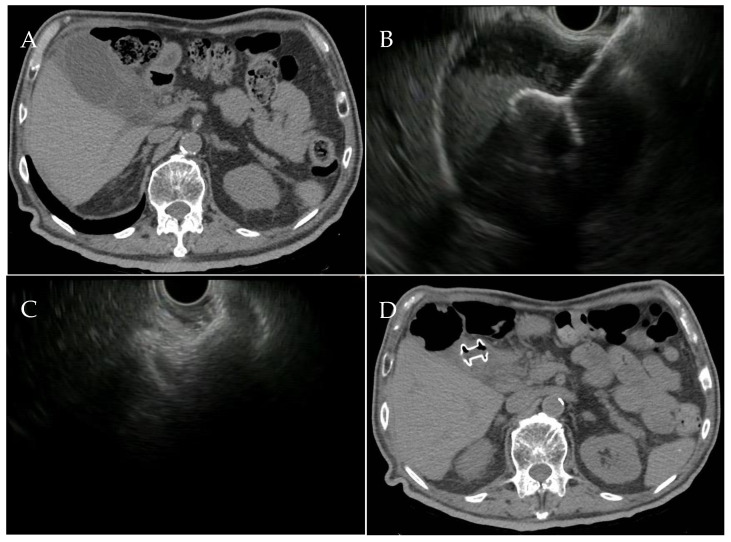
(A) CT scan image of an acute cholecystitis. (B) EUS images of the LAMS deployment and (C) the fully deployed LAMS into the gallbladder. (D) CT scan image showing the drained gallbladder with the LAMS in situ. CT: computed tomography; EUS: endoscopic ultrasound; LAMS: lumen-apposing metal stent.
